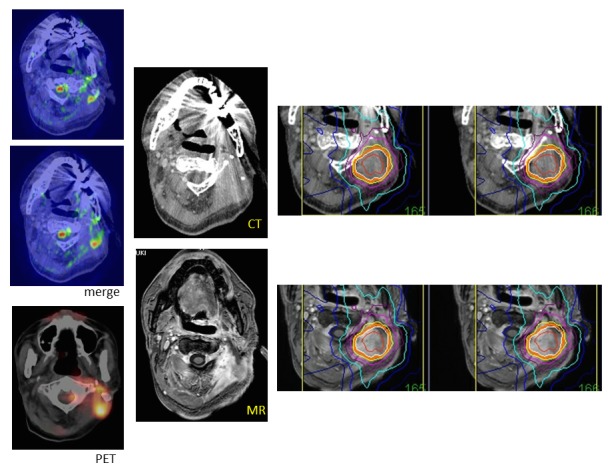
A recurrent neck cancer. MR diffusion images merged to thin-sliced CT axial sections showing a recurrent lesion in the neck, in the same locations as indicated by the PET scan (left line). The Gd-enhanced MR image is fused to CT and used for dose planning (center). The target of hypofractionated radiotherapy is demarcated based on the merged images.
